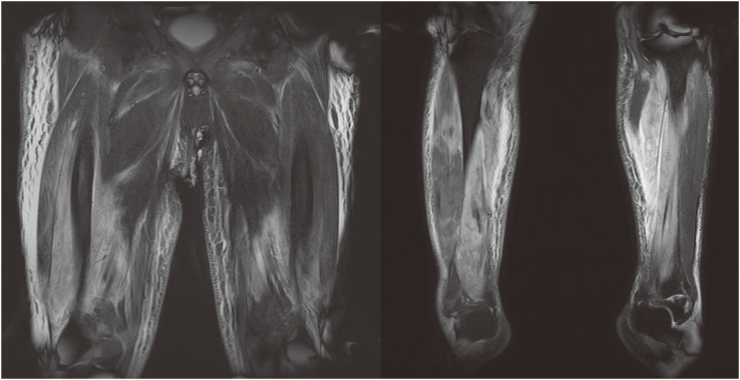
Magnetic resonance imaging of both lower extremities shows multiple high-signal areas in the muscles of the bilateral thighs and lower legs.
